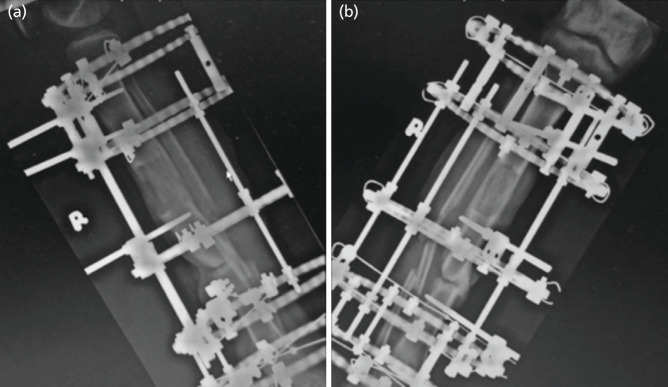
Radiograph of right tibia fibula, (a) lateral view and (b) anterior posterior view.
